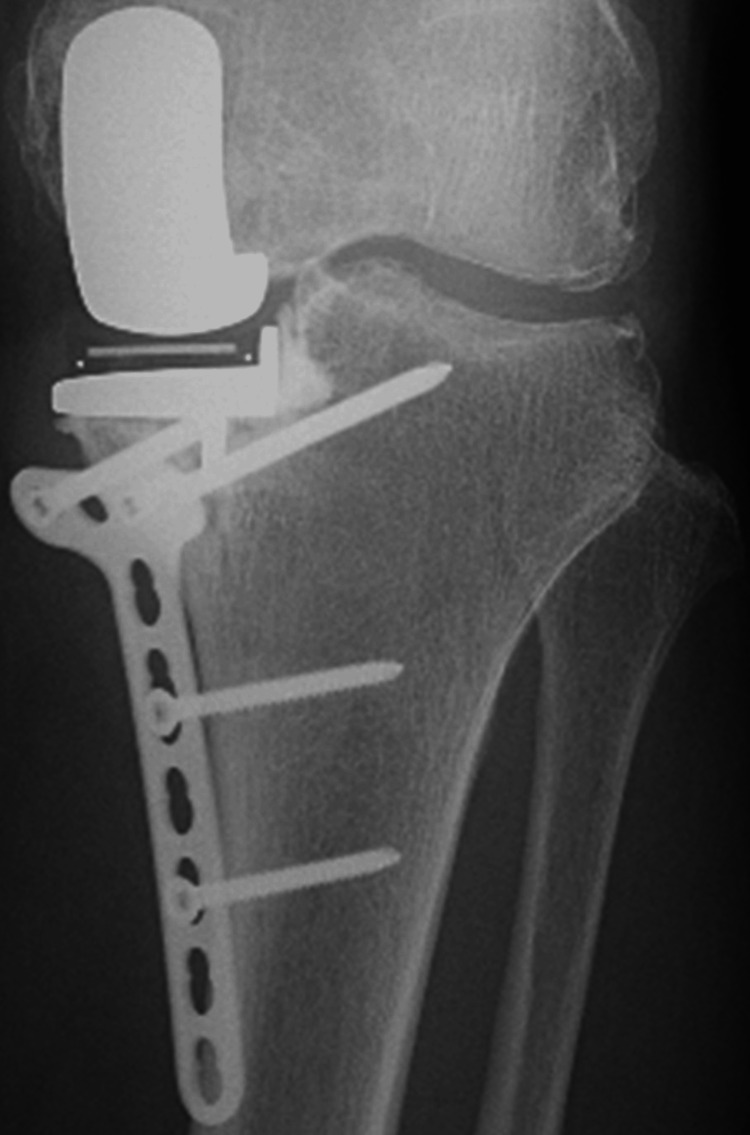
Radiography of reoperation with tibial compression plate (AP view)
